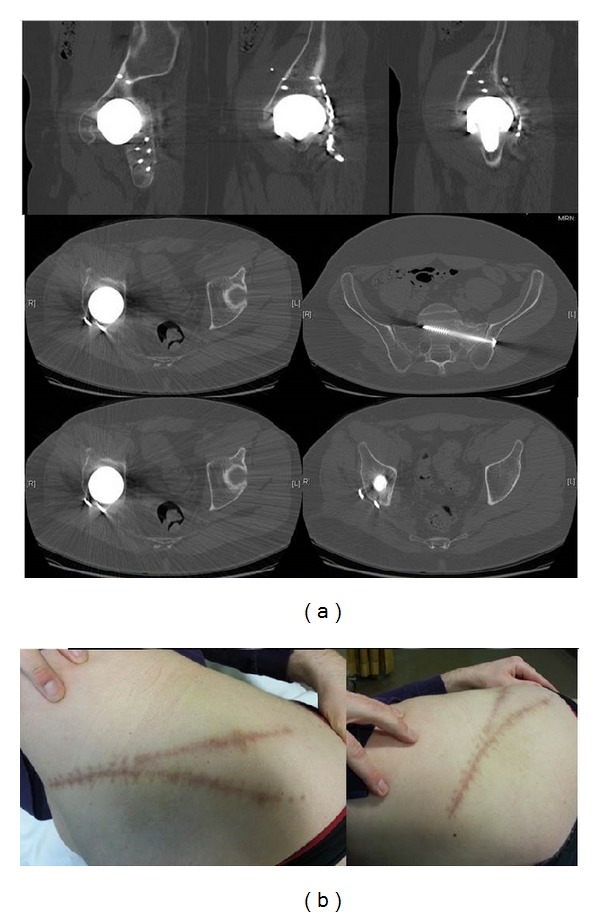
Clinical wound condition and CT-scan result 1 year postoperatively.
